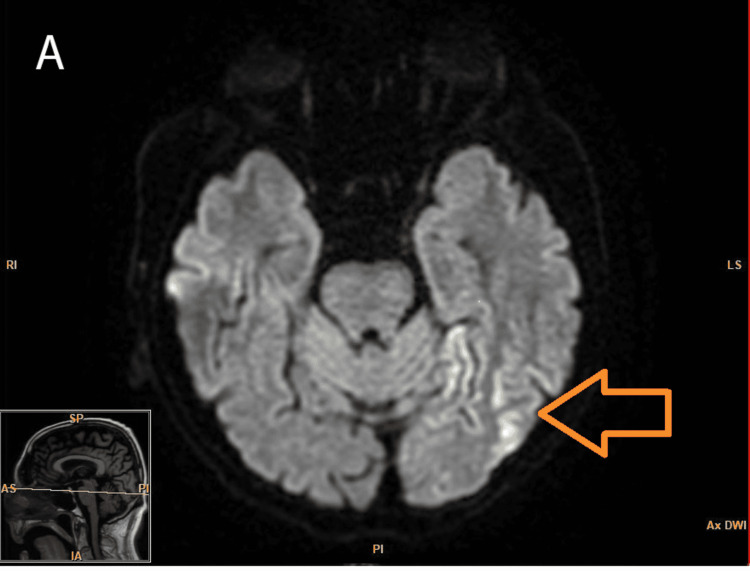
Axial diffusion-weighted MRI showing hyperintensities Bilateral cortical high signal intensities, more prominent on the left side (arrow)
